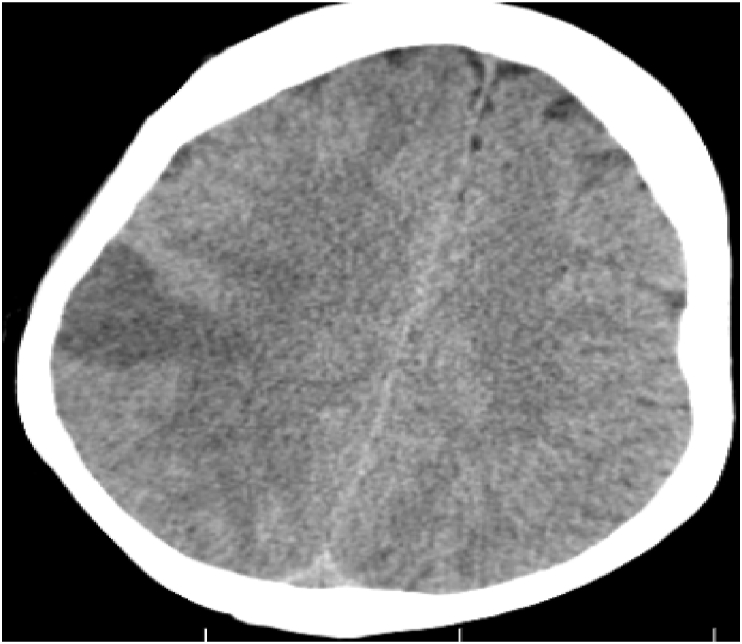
Brain CT shows right parietal infract.
